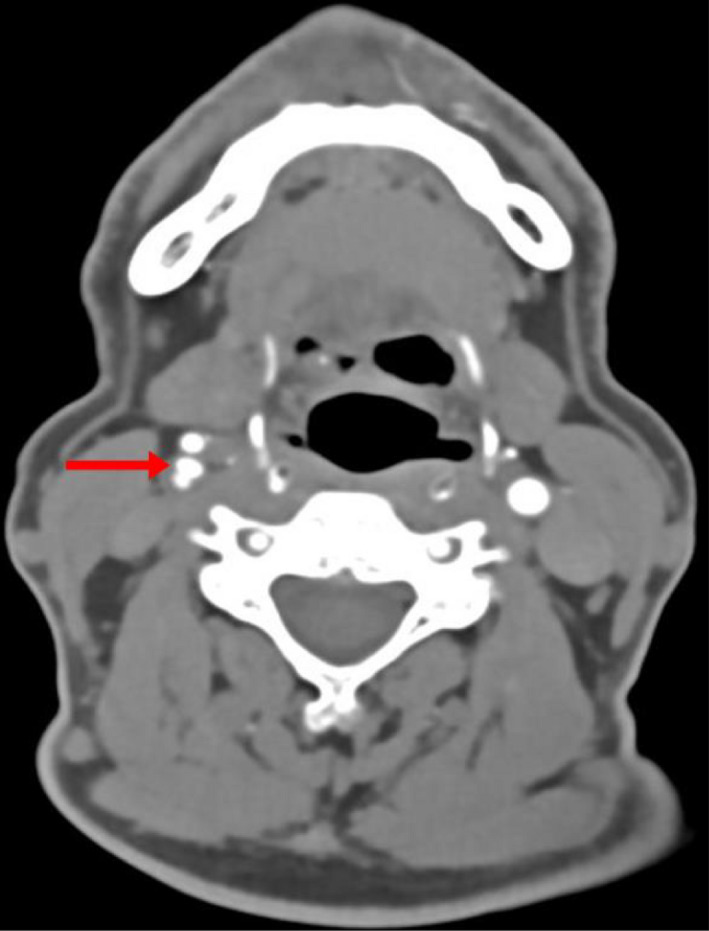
CTA of the neck, axial sections showing a triple lumen sign just distal to the right common carotid artery bifurcation. The normal external carotid artery (ECA) anteriorly and the ICA posteriorly divided into two separate lumens with fine line
